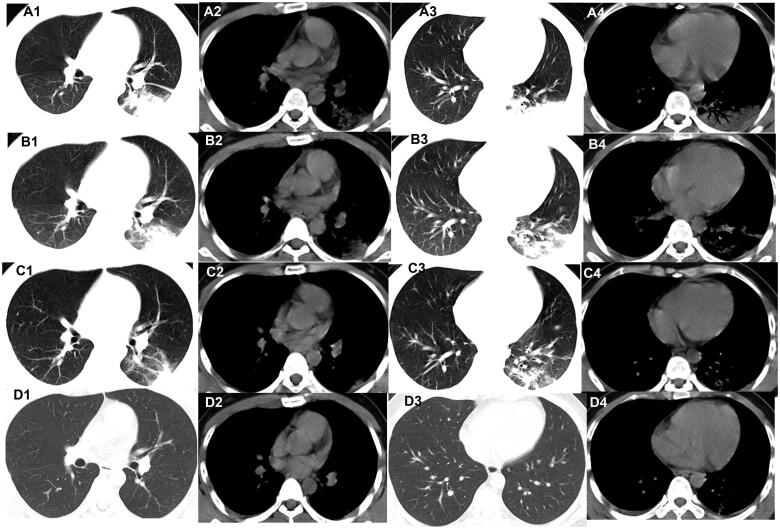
Evolution of consolidationon chest CT of patients with COVID-19. A 45-year-old male Wuhan resident presented with fever and cough for 3 days. (A1–A4) The first non-contrast-enhanced chest CT revealsconsolidation and air bronchogram in the left lower lobe (initial chest CT). (B1–B4) Follow-up chest CT 4 days after the first shows that both the scope and density of the lesions decrease (stage I*). (C1–C4) Follow-up chest CT 8 days after the first shows that both the scope and density of the lesions decrease further (stage II*). (D1–D4) Follow-up chest CT 12 days after the first shows that the lesions are almost absorbed completely (stage III*). *The stage does not represent the course of COVID-19 but the time interval between the two adjacent CT scans.
